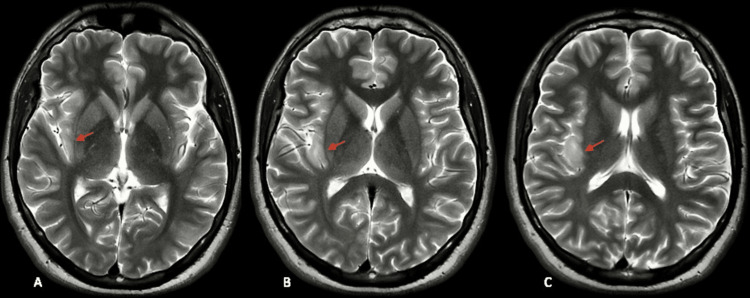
T2-weighted axial MRI images showing hyperintense right posterior insular focal cortical dysplasia MRI: magnetic resonance imaging
